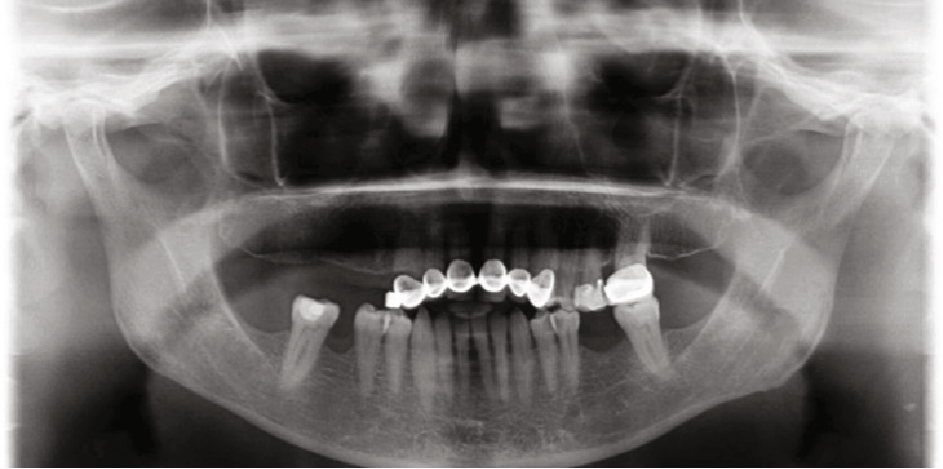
Posterior edentulous areas in the panoramic radiograph.
